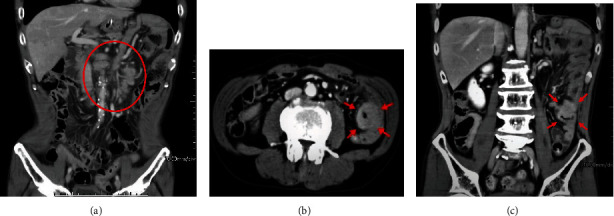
Axial and coronal images of multidetector row CT scan. (a) The preoperative CT scan showed swollen para-aortic and mesenteric lymph nodes. These were diagnosed with lymphatic metastases from the primary esophageal carcinoma. (b, c) CT scans revealed a single occlusive point. The circumferential tumor let us take into consideration an esophageal cancer metastasis as a potential cause of the obstruction. The arrows show a thickened small intestine wall, thus showing the metastatic site.
